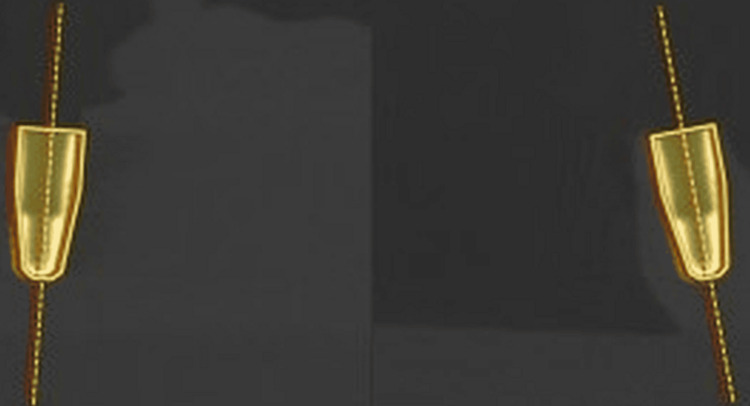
Post-implant placement CBCT scans CBCT: Cone-beam computed tomography
